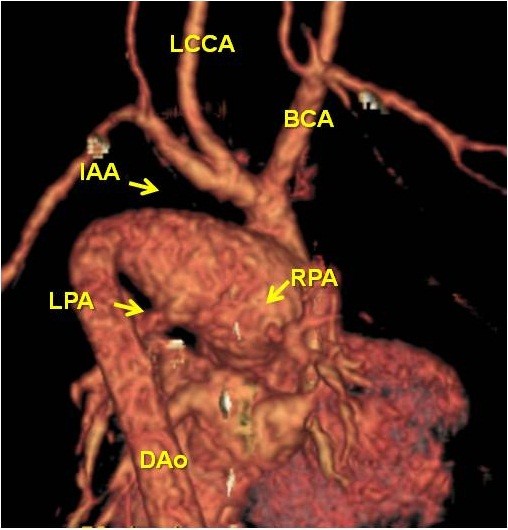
Posterior 3D CT view. IAA type A and RPA bifurcated distally to LPA were observed.
